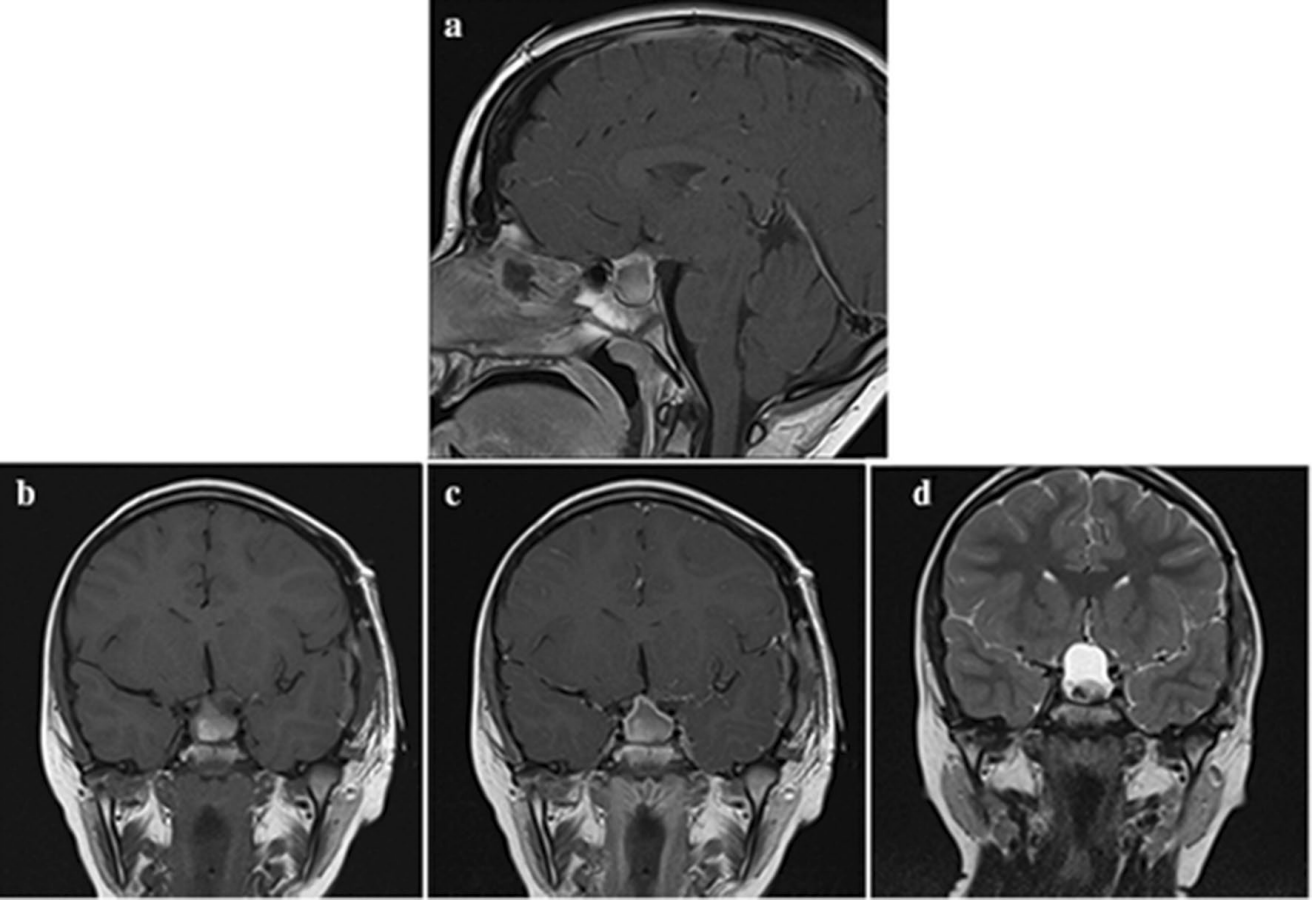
Case 5—Exemplar MR imaging findings in pituitary xanthogranuloma. There is a cystic lesion demonstrating peripheral rim contrast enhancement, and a tumour epicentre identifiable in quadrant 1. a T1 weighted sagittal (post-contrast); mixed intensity lesion with predominantly high T1W signal centrally, and peripheral rim enhancement. b T1 weighted coronal (pre-contrast); mixed intensity lesion with predominantly high T1W signal centrally. c T1 weighted coronal (post-contrast); mixed intensity lesion with predominantly high T1W signal centrally. The peripheral rim enhancement is easily appreciated in this image. d T2 weighted coronal; mixed intensities within the lesion, suggestive of the presence of both solid and cystic components
